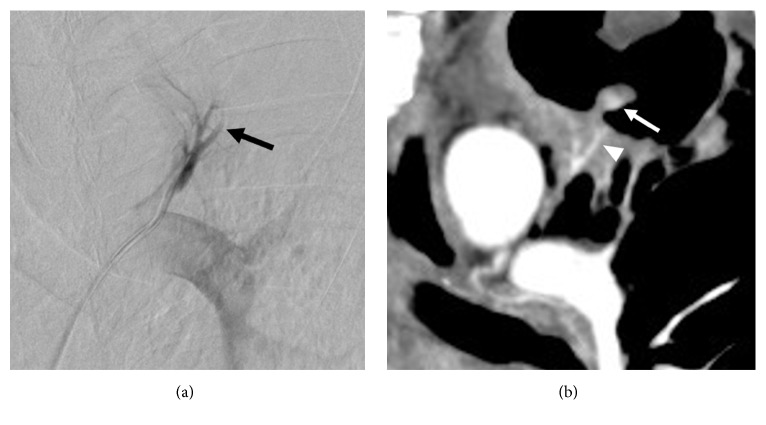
Comparison between pulmonary angiography and computed tomography image. (a) Left segmental pulmonary angiography shows abrupt tapering of the arterial branch (arrow), which indicates retrograde flow from the bronchial to the pulmonary artery. (b) Contrast-enhanced coronal computed tomography image shows the aneurysm (arrow) and the parent artery of the aneurysm (arrowhead). The parent artery corresponds to the abrupt tapering of the arterial branch, which was seen in the left segmental pulmonary angiography.
